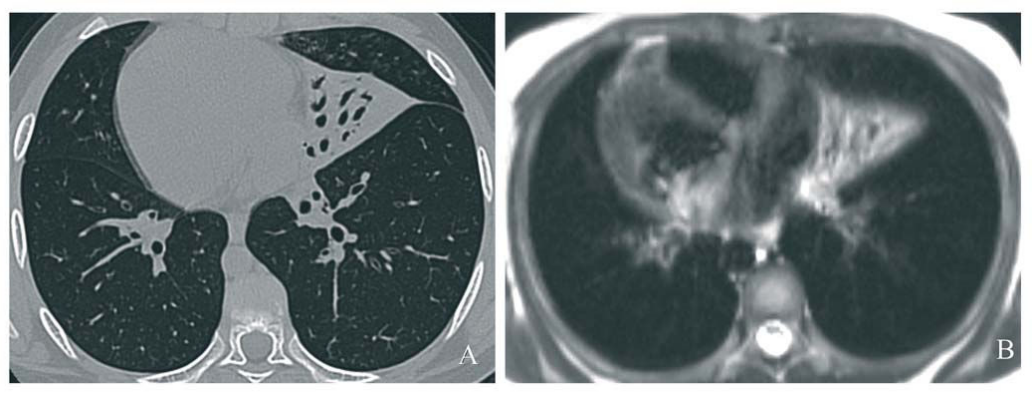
Transversal CT image (A) and transversal MRI HASTE (B) sequence of a 10.4-year-old boy with Kartagener syndrome. The scans demonstrate an area of consolidation in the middle lobe.
